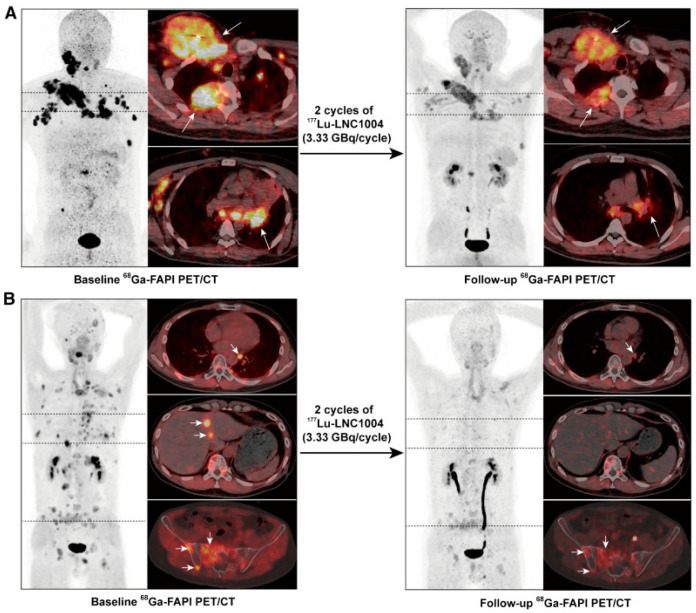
Two cases with partial response after two cycles of 3.33 GBq [^177^Lu]Lu-EB-FAPI. At baseline, ^68^Ga-FAPI PET/CT shows high FAP expression in metastatic lesions (bone, lymph nodes, liver) in two patients with disease progression after TKI treatment for RAI-R thyroid cancer. (A): a 36-year-old man with high uptake in metastatic lymph nodes and bone metastases (left, arrows). (B): a 42-year-old man with high uptake in bone, liver and lymph node metastases (left, arrows) Follow-up ^68^Ga-FAPI PET/CT indicates a favorable response with reduced FAPI uptake and size reduction (right panels, arrows). Figure reproduced from Fu et al., Fibroblast Activation Protein-Targeted Radioligand Therapy with 177Lu-EB-FAPI for Metastatic Radioiodine-Refractory Thyroid Cancer: First-in-Human, Dose-Escalation Study, Clin Cancer Res. 2023 Dec 1, doi: 10.1158/1078-0432.CCR-23-1983. [19] under CC BY 4.0 (https://creativecommons.org/licenses/by/4.0/, accessed on 3 September 2025).
